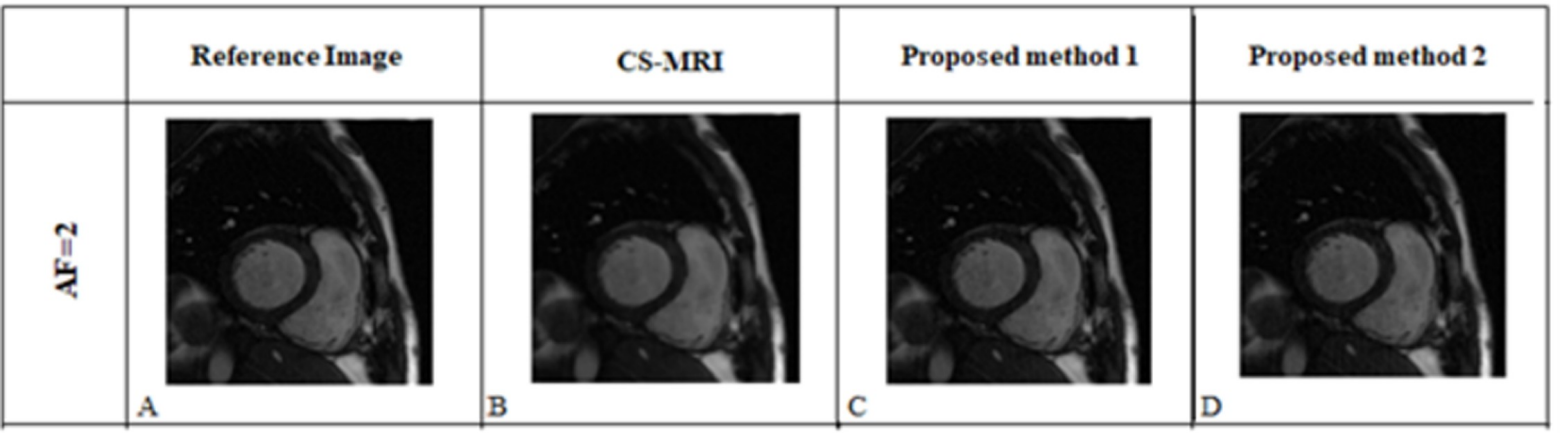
Reconstructed cardiac images of CS-MRI, proposed methods 1 and 2 for clinical dataset (i.e., 18 receiver coils human cardiac data). Column ‘A’ presents the reference image which is obtained by taking the adaptive coil combination [20] of the fully sampled 18 receiver coils human cardiac data. Columns ‘B-D’ show the reconstructed images of CS-MRI, Proposed Methods 1and 2, respectively.
